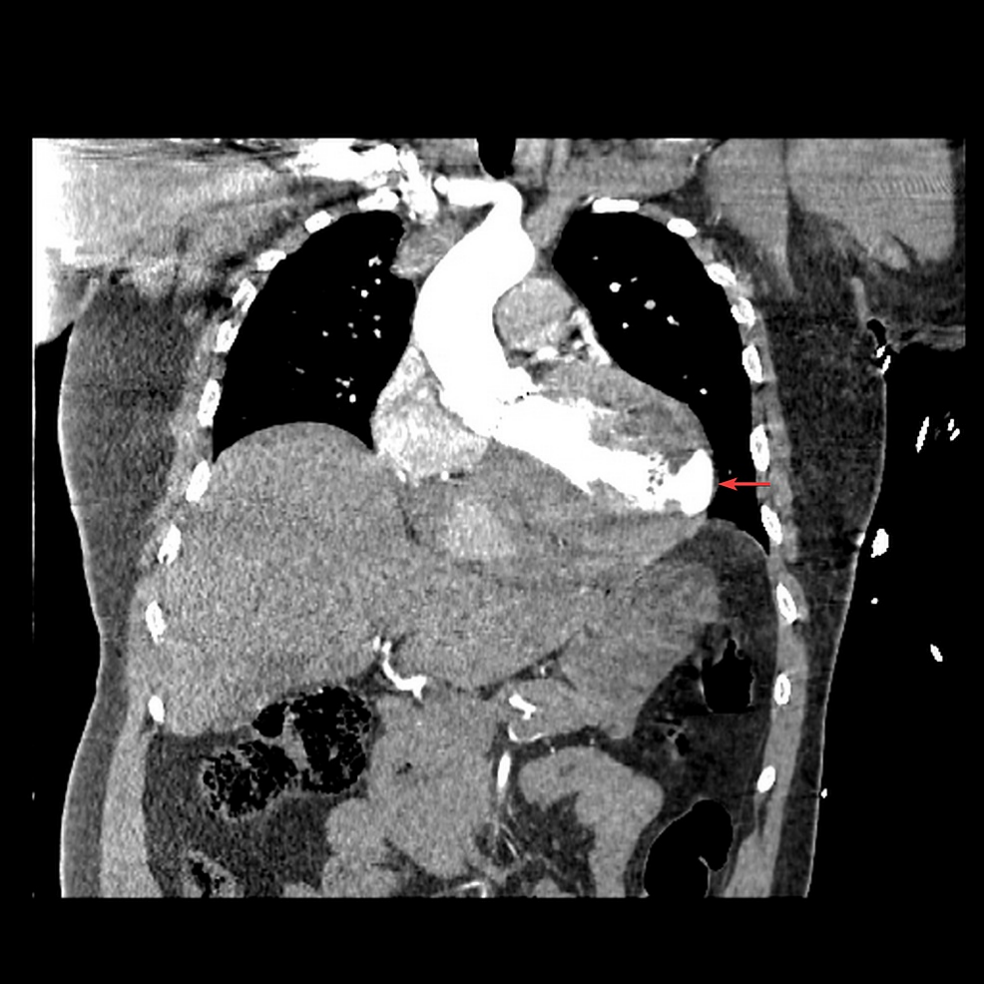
Coronal view of computed tomography with angiography of the chest showing a ventricular free wall rupture with extravasation of contrast from the left ventricle to the pericardial space (red arrow).
